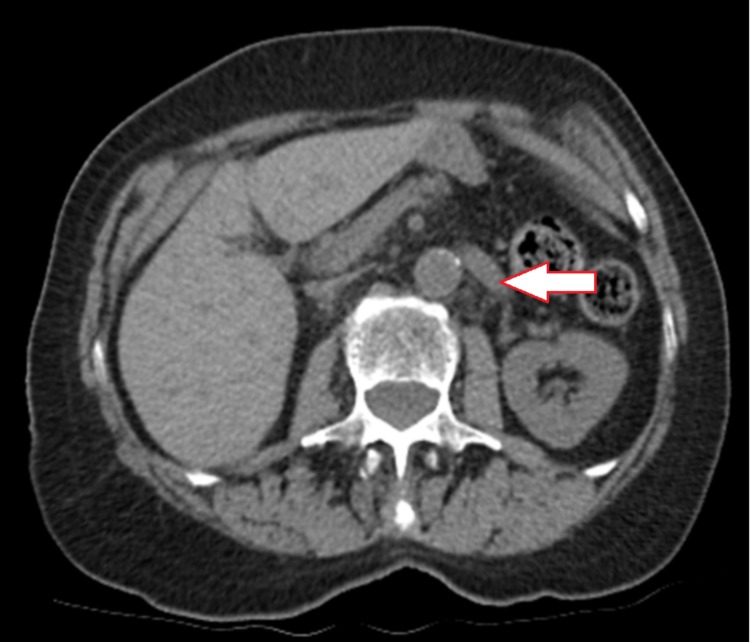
Computed tomography (CT) demonstrating resolution of lymphadenopathies after eight cycles of systemic therapy
